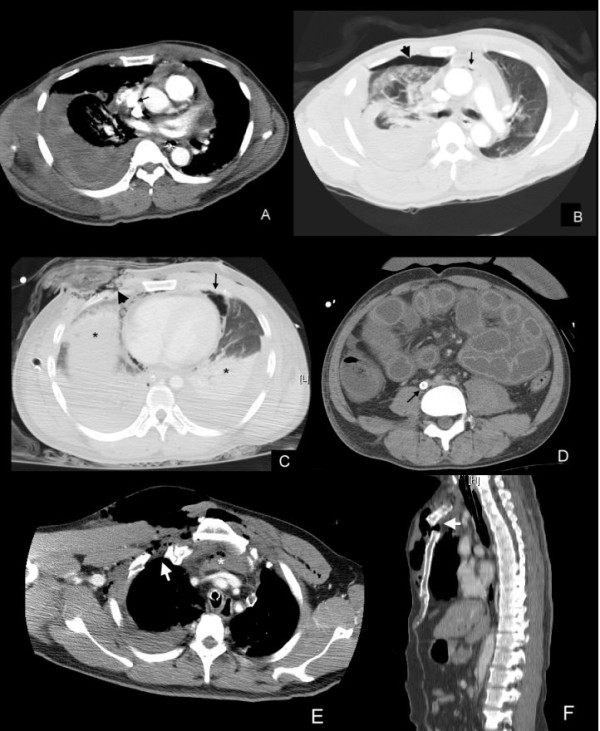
Special computed tomographic images. Blunt chest trauma: Hemothorax from a lacerated right atrium accompanied with a ruptured pericardium (Case 3). A. A massive right-sided hemothorax accompanied with a hemopericardium. The right auricle is surrounded by thrombus without extravasations of the contrast media into the right hemithorax (arrow). B. The coexistence of a right-sided pneumothorax (arrowhead) and a pneumopericardium (arrow) indicates a right-sided pericardial disruption in this case. Injuries in a victim of high-voltage electrocution (Case 5): C. An open pneumothorax with a defect of the right chest wall (arrowhead). Pulmonary consolidations (*) and pneumomediastinum (arrow) are also presented. D. The “shock bowel” appearance. An ECLS cannula is in the inferior vena cava (arrow). Blunt chest trauma: A flail chest from injuries of the sternoclavicular articulation (Case 8). E. A comminuted fracture in the proximal segment of the right clavicle (arrow). Significant soft tissue damages with a retrosternal hematoma (*) is presented. F. A displaced fracture of the manubrium. The cardiac displacement and significant subcutaneous emphysema here are caused by a right-sided tension pneumothorax identified in other views (arrow).
